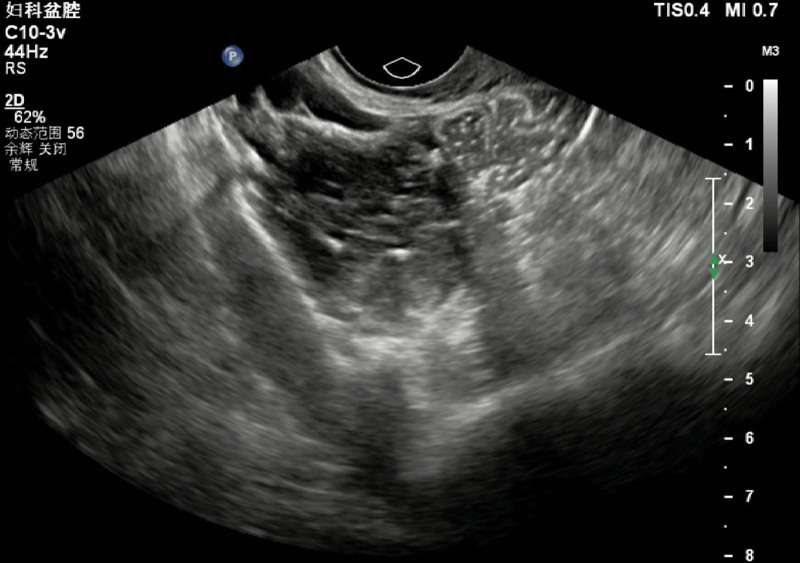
Ultrasonography pictures.
